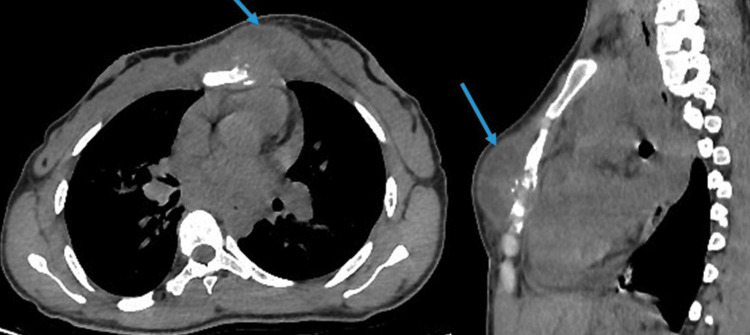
Axial (A) and sagittal (B) sections of the chest CT scan Images showing a left-sided presternal mass (blue arrow) with osteolytic lesion in the sternum (arrow)
